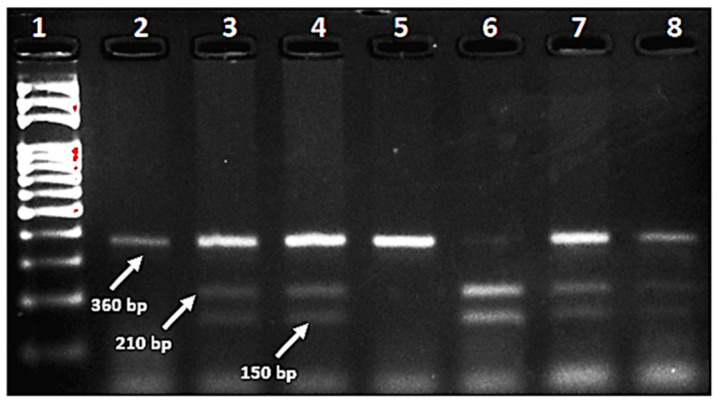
C677T genotypes of the MTHFR gene identified by the PCR-RFLP method. Lane-1: DNA Ladder (100 bp). Lane-2, 5: Wild-type CC. Lane-3, 4, 7, 8: Heterozygous CT. Lane-6: Homozygous mutant TT.
